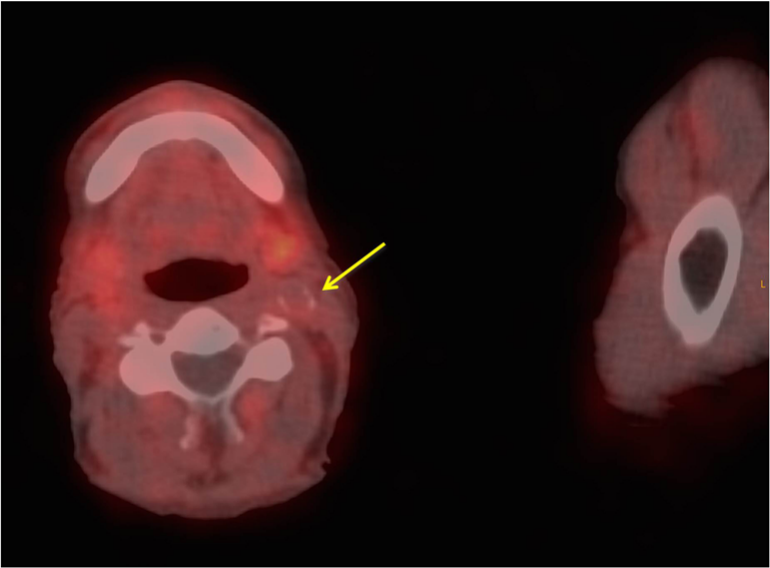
Fused PET/CT images show only slight ^68^Ga-DOTATATE uptake at the bifurcation of the left carotid artery with, on the CT images, as part of the fused PET/CT images, visible calcification (*arrow*). Combined molecular (^68^Ga-DOTATATE PET) and morphological imaging (CT) indicates more stable, chronic arterial vessel wall changes
